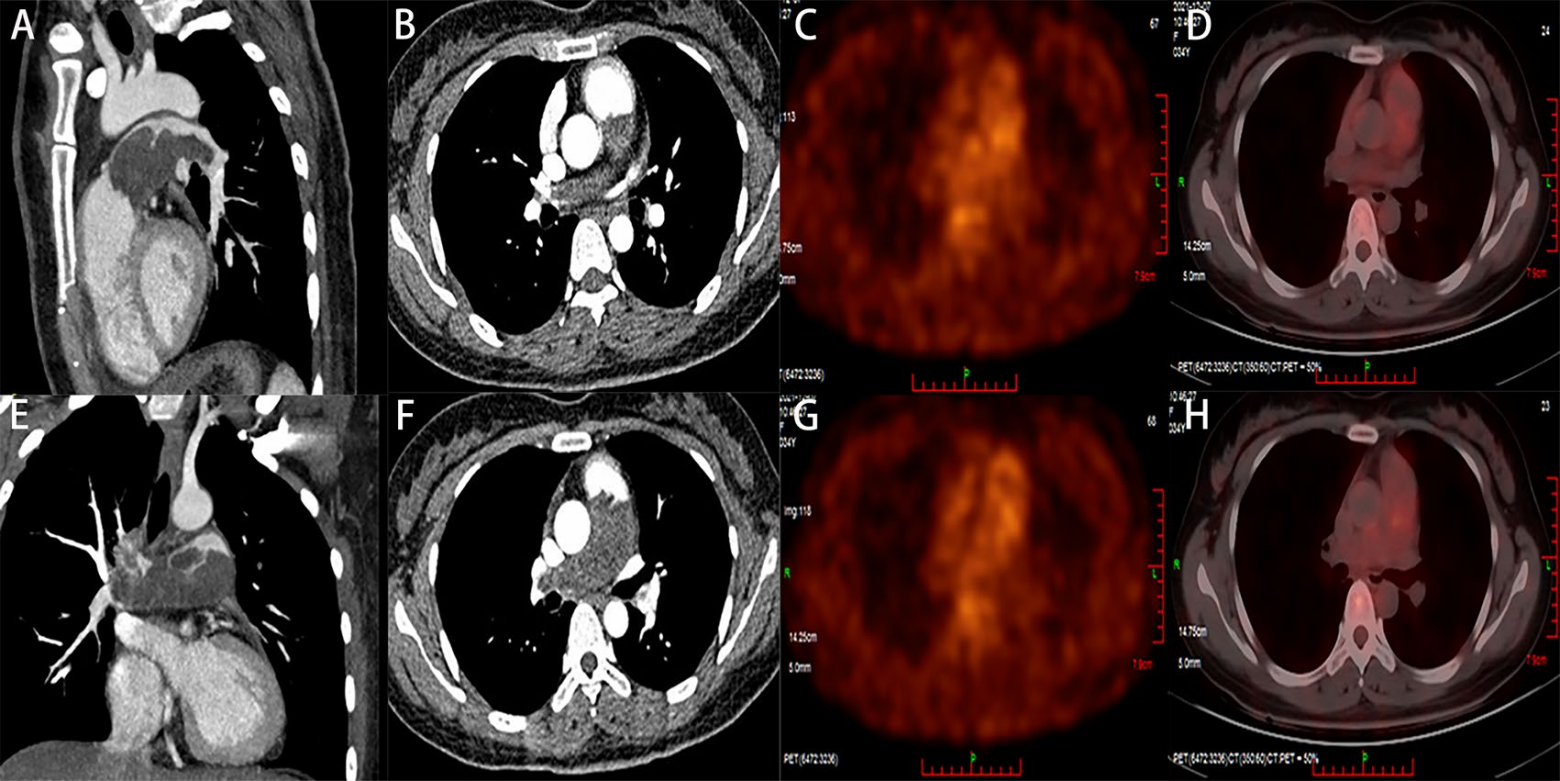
A, B, E, F Preoperative CTPA: The dilation of the pulmonary trunk, filling defects in the main pulmonary artery, left and right pulmonary arteries. C, D, G, H PET/CT: a slight increase in glucose metabolism of the mass
